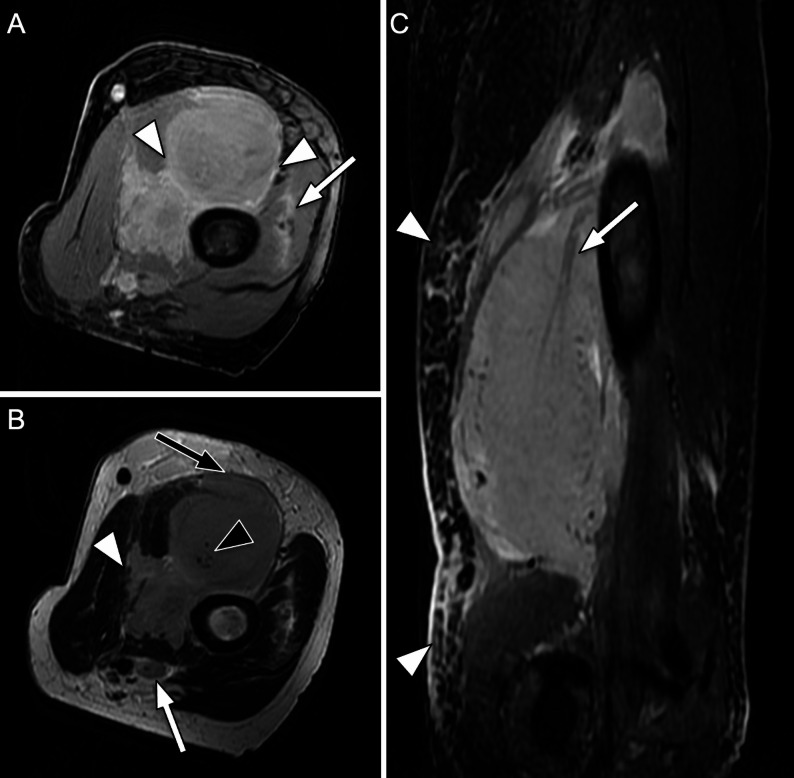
MRI of a 73-year-old male presenting with non-Hodgkin lymphoma manifestation in the upper arm. A Transversal contrast enhanced fat-saturated T1-weighted image shows partial peripheral enhancement (arrowheads) accompanied by slightly less intense homogenous enhancement of the remaining tumor. Moreover, one can note manifestation in the extensor compartment, encasing branches of the medial collateral artery (arrow). B Transversal T2-weighted image shows encasement of radial nerve and collateral radial artery (black arrowhead) and close association of an additional lesion to the brachial neurovascular bundle (white arrow). Moreover, co-existence of well-defined (black arrow) and ill-defined (white arrowhead) tumor margins can be recognized. C Coronal STIR image shows subcutaneous stranding (arrowheads), long segmental involvement and longitudinally traversing deep brachial artery (arrow)
